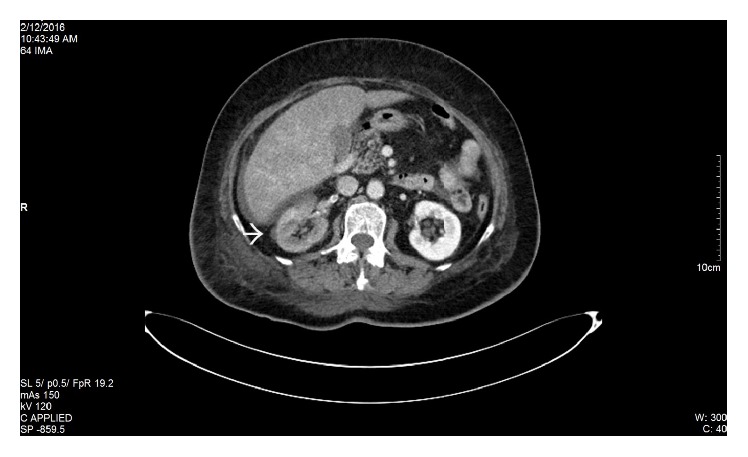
Contrast-enhanced CT scan. Decreased uptake of contrast in the right kidney. The white arrow shows the right kidney with small areas of contrast uptake suggestive of a partial occlusion of the right renal artery.
